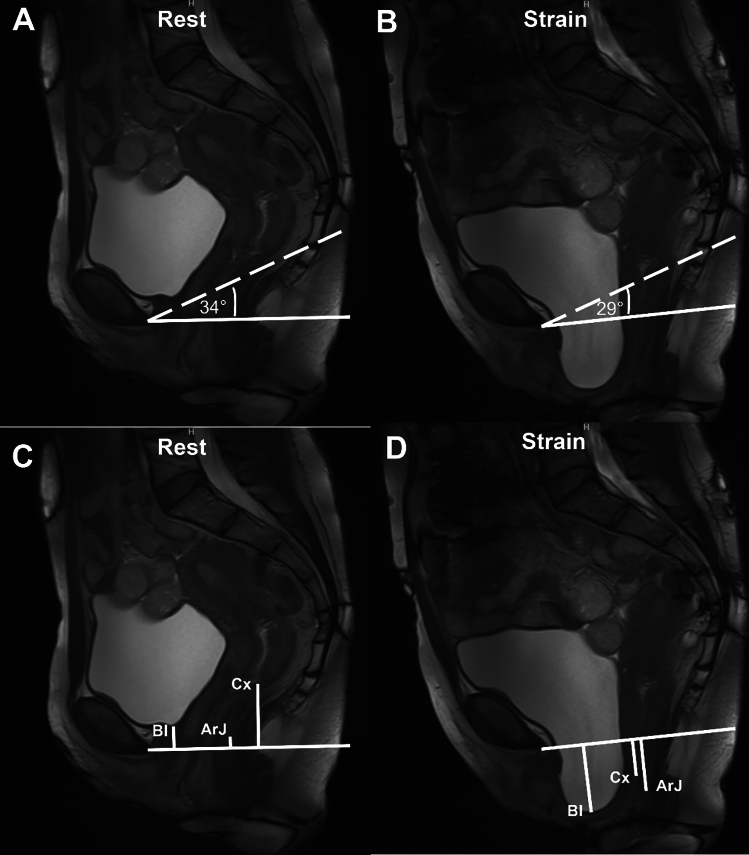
38-year-old (gravida 2, para 2) patient with multi-compartment organ prolapse presenting with symptoms of vaginal bulge and stress urinary incontinence. Top row depicts dynamic midsagittal MR images showing the position of the PICS line at rest (A) and straining (B) adjusted for differences in pelvic inclination. The interrupted line represents the sacrococcygeal-inferior pubic point (SCIPP) line which connects the sacrococcygeal joint to the inferior border of the pubic symphysis. The PICS line (solid line in A–D) is then drawn from the inferior border of the pubic symphysis with a 34° and 29° clockwise rotation from the SCIPP line at rest and straining, respectively. Bottom row (C, D) shows organ point measurements with reference to the PICS line in this patient at rest (C) and straining (D). At rest, the bladder (Bl), cervix (Cx), and anorectal junction (ArJ) are located at 1.5 cm, 4.2 cm, and 0.9 cm above the PICS line. At straining, the bladder descents to 4.0 cm below, the cervix to 1.9 cm below, and the anorectal junction to 3.1 cm below the PICS line. Measurements are made perpendicularly to the PICS line. Clinically, the patient was graded as POP-Q III° for the anterior, POP-Q II° for the middle, and POP-Q I° for the posterior compartment
